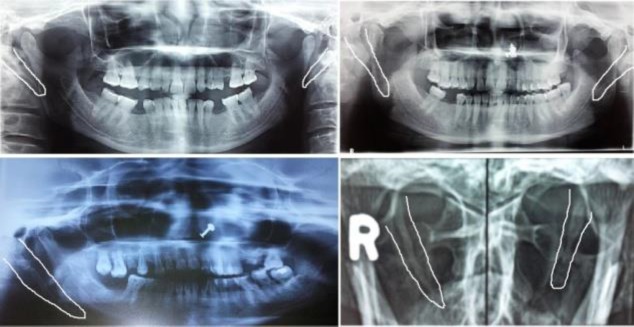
Multiple X-ray views showing elongated styloid process
